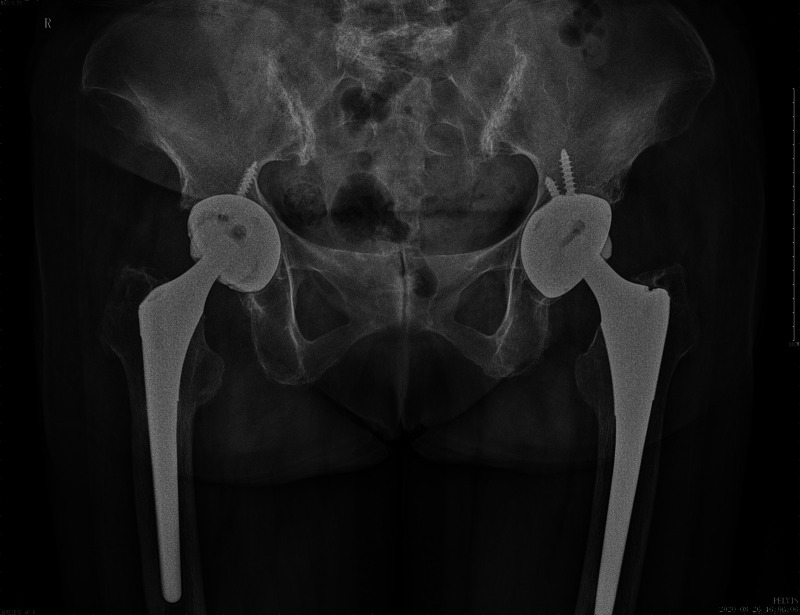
Postoperative X-rays of hip joints showed well integrated and positioned component.
